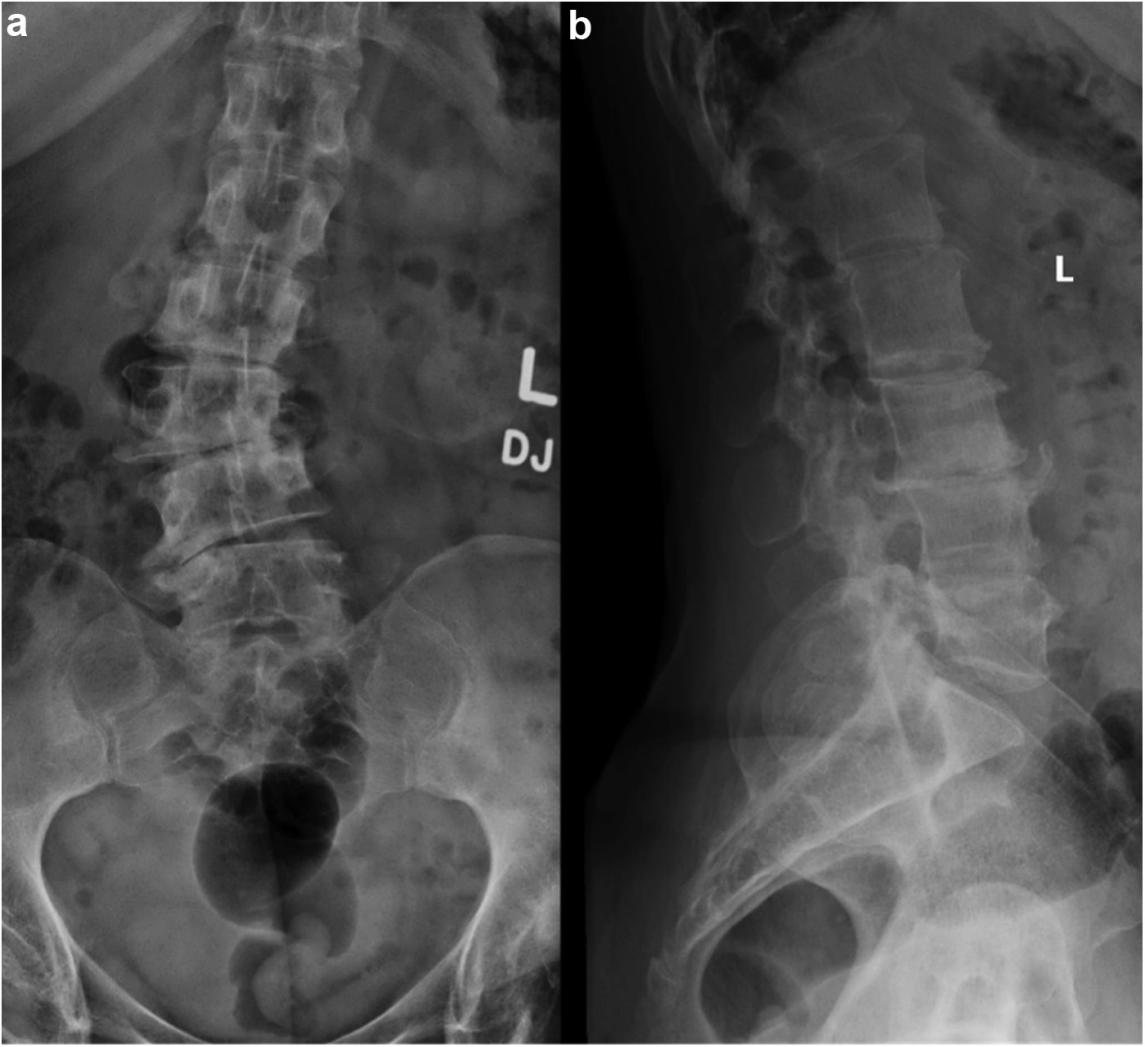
Preoperative anteroposterior (a) and lateral (b) radiographs of the lumbar spine demonstrating degenerative lumbar scoliosis.
